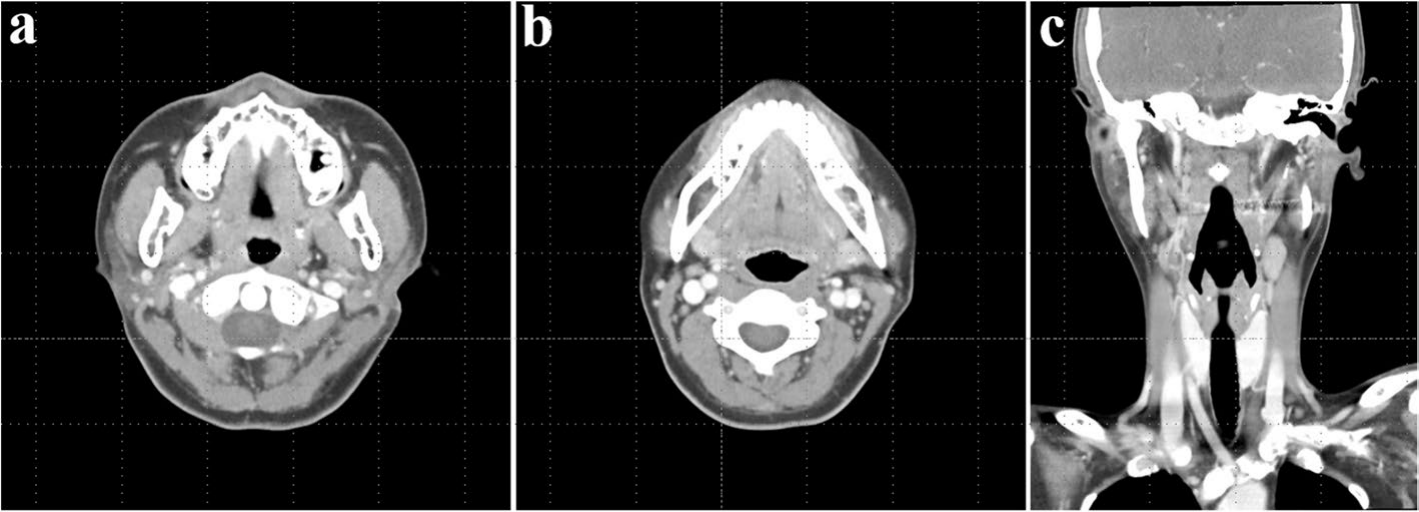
Postoperative CT images at 4 months. a Axial view (parotid gland area). b Axial view (lymph node area, level II, III); no pathologic lymph nodes. c Coronal view
